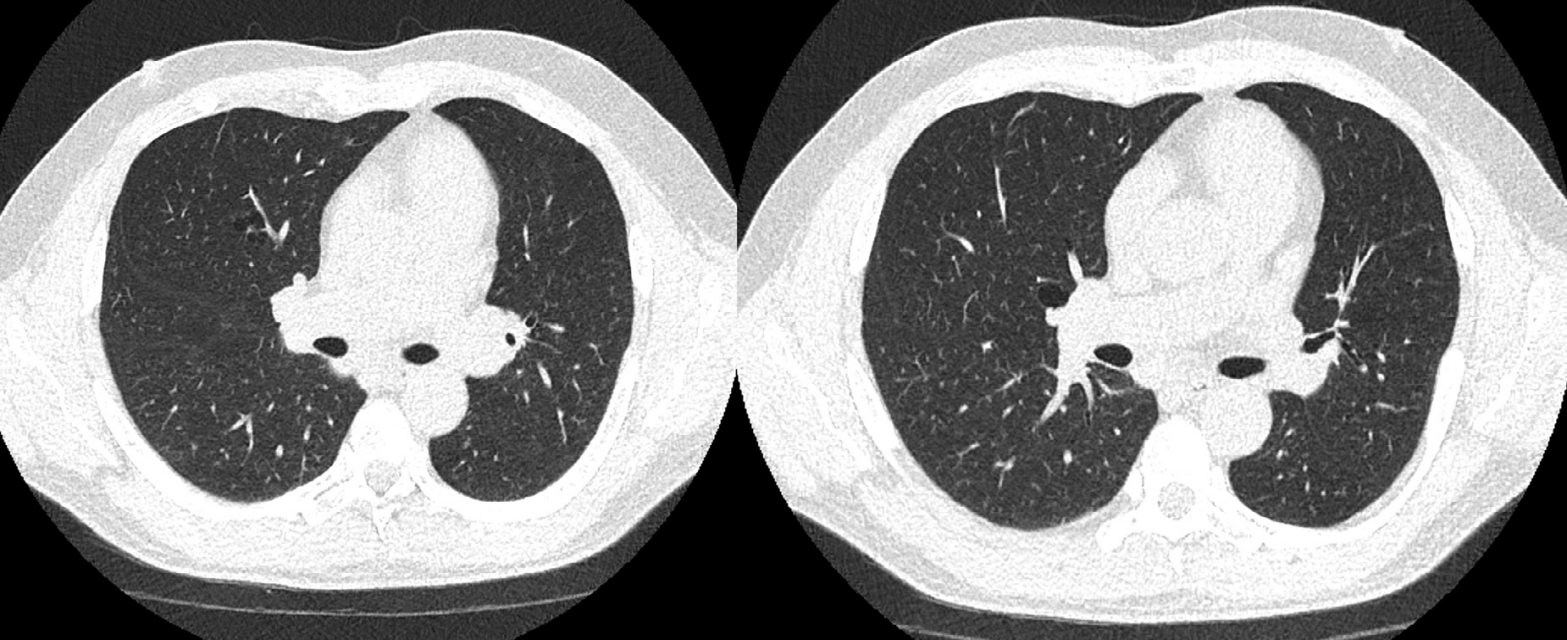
CT Chest without contrast: Axial images demonstrate multiple thin-walled elliptical well-defined para-mediastinal cysts in a basilar distribution with preserved lung volume and no evidence of interstitial lung disease
